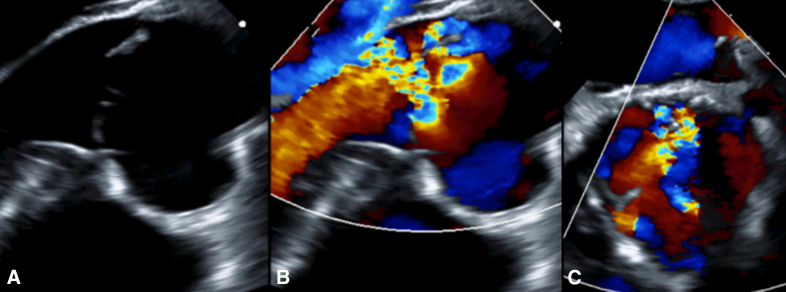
Preoperative echocardiogram demonstrated severe aortic regurgitation in long-axis (A, B) and short-axis views (C).
